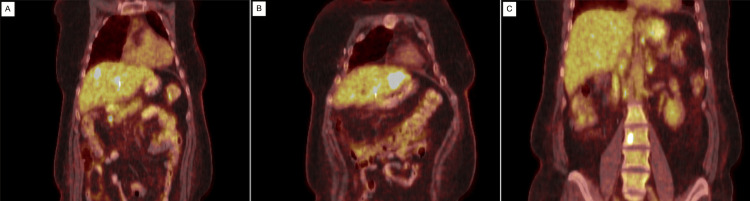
Full-body PET CT scan performed during the second hospital admission demonstrating multiple hypermetabolic lymph nodes in the chest and abdomen (A), as well as multiple hypermetabolic hepatic (B) and spinal (C) lesions. PET: Positron Emission Tomography; CT: Computed Tomography
